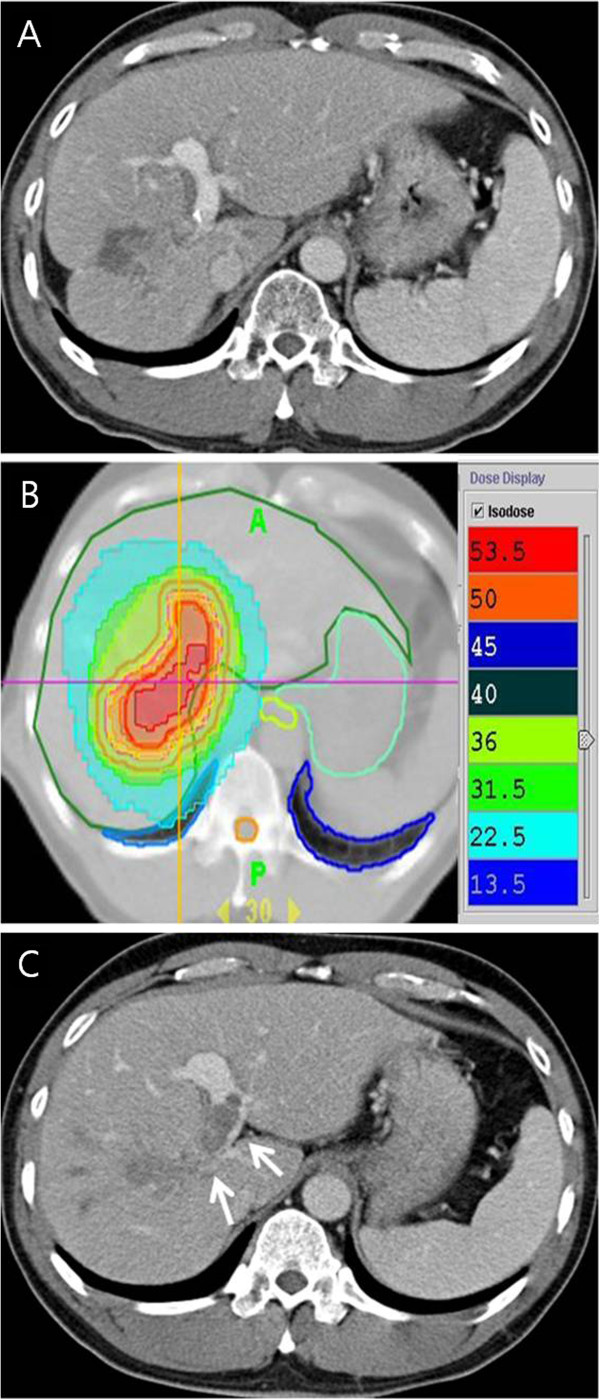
Case presentation of a patient with PVTT treated with concurrent chemoradiotherapy with HT (50 Gy/10 fractions/2 weeks) and daily administration of capcitabine during radiotherapy. (A) A contrast-enhanced CT in the portal phase shows the diffuse infiltrative HCC with right and main PVTT (white arrows). (B) The axial dose distribution of HT with effectively covering the GTV and sparing the adjacent normal organs (C) One month after treatment, recanalization of the venous obstruction due to PVTT was achieved.
